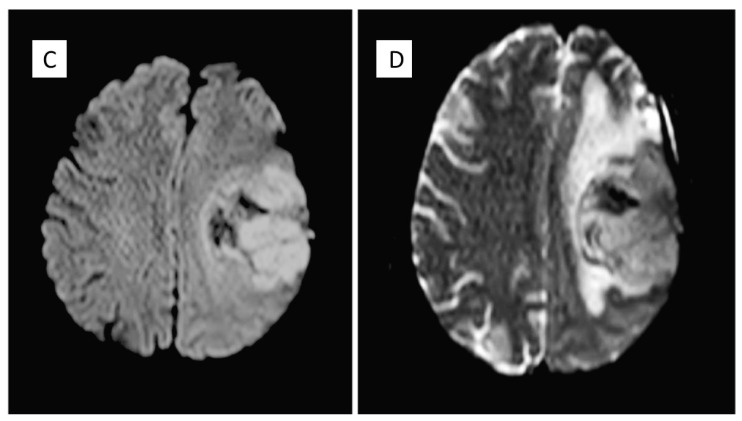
(A,B) Axial and coronal projections. Large meningioma in the left parietal area of 5 × 5 cm. The mass is surrounded by perifocal oedema with consecutive compression and dislocation to the right of the ventricular system. The temporal horn of the ipsilateral ventricle results in communication with the subarachnoid space. The administration of the gadolinium determines inhomogeneous accentuation of the mass. (C,D) PM displays an heterogenous hyperintensity in DWI surrounded by an hypointense oedema.
